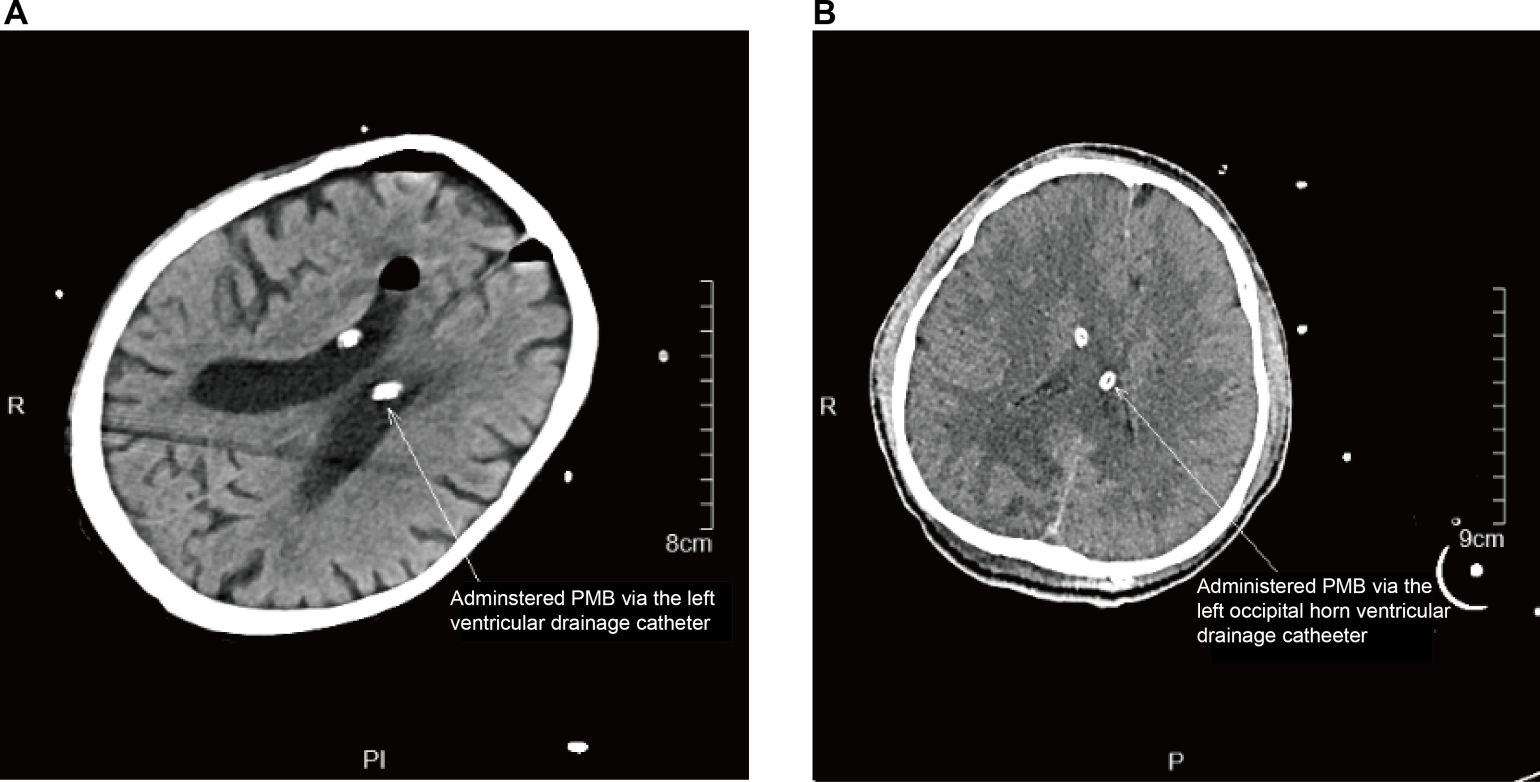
Head CT images of patients. (A) Head CT images of patient 1. The patient had EVDs placed in both lateral ventricles. PMB was administered through the left EVD from day 1 to day 3. On days 4 and 6, CSF samples were simultaneously collected through the bilateral ventricular drainage catheters to measure PMB concentrations in the CSF. (B) Head CT images of patient 2. Ventricular drainage catheters were placed in both the left occipital horn and the right frontal horn. PMB was administered via lumbar puncture on days 1 and 2, and through the left occipital horn drainage catheter on days 3 and 4. On day 5, after IVT administration, the drainage catheters were clamped for 1 hour, and CSF samples were collected 2 hours later from both the left occipital horn and right frontal horn drainage catheters.
